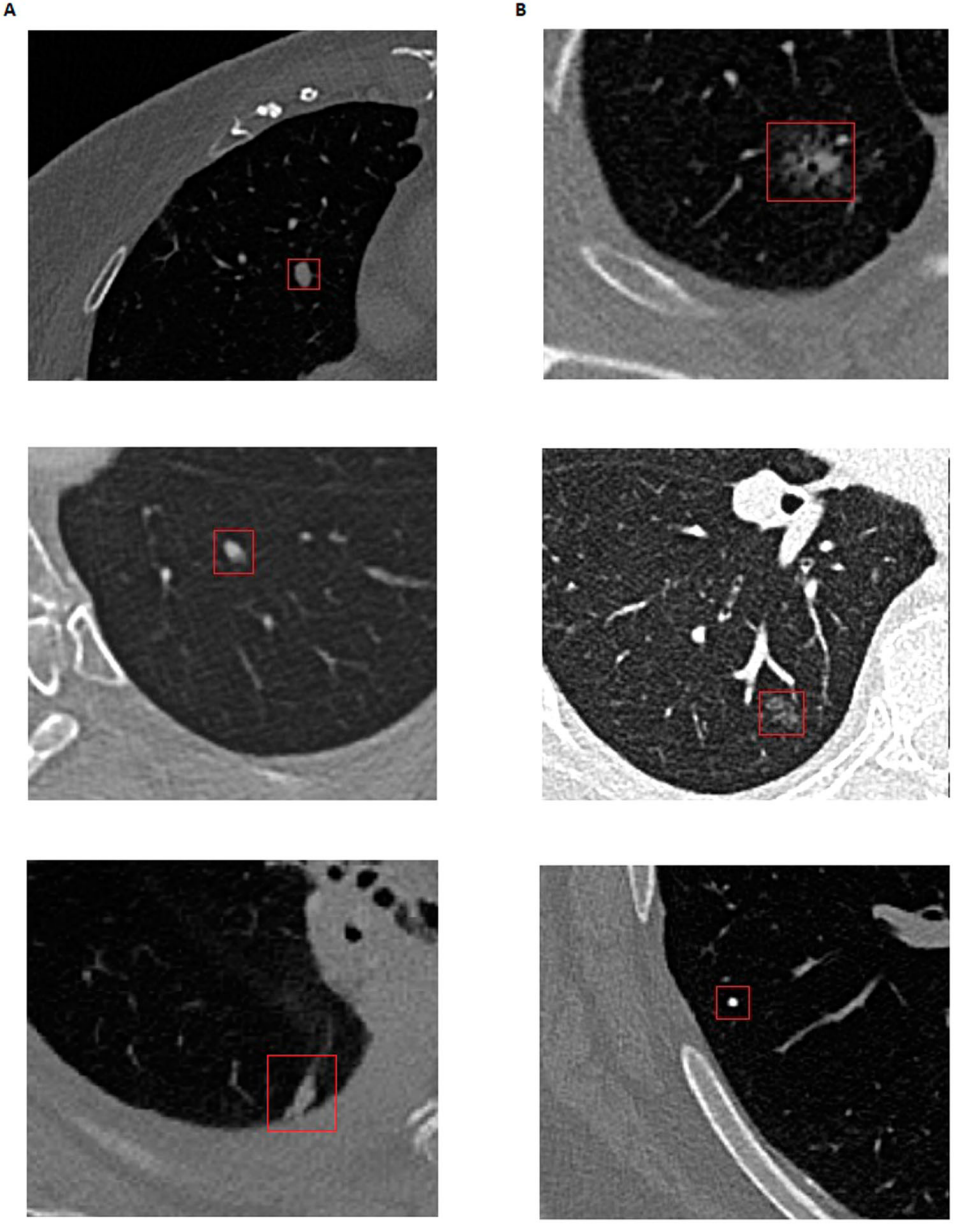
Examples of FP and FN findings: On the left (A), three examples of false positives (FP) are depicted. According to our reviewing radiologists, the top one represents an obvious blood vessel in the middle lobe; the middle CT scan contains slight motion artefacts, likely resulting in the incorrect flagging of a blood vessel in the left lower lobe; the bottom one is a perifissural lymph node. On the right (B), three false negatives (FN) are shown, these were missed by the CAD-system. The top image shows a part-solid nodule in the right lower lobe; the middle image displays a ground-glass nodule, also located in the right lower lobe; the bottom image features a subtle, calcified solid nodule situated in the right lower lobe
